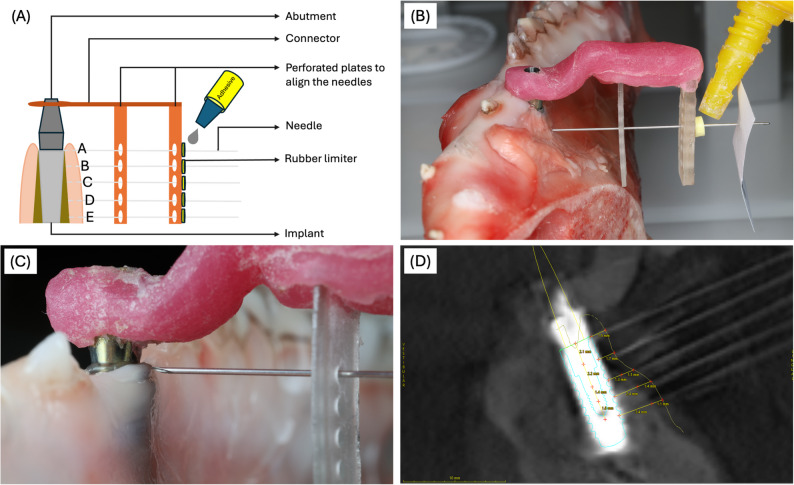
Mucosal thickness measurement using transgingival probing and cone*-*beam computed tomography. A Measurement device for transgingival probing (TP) and cone‑beam computed tomography (CBCT) with reproducible horizontal and vertical alignment at standardized points (A–E). B Fixation of the rubber limiter. C Needle in contact with the mucosa at point A. D Superimposition of optical scan and CBCT with mucosal thickness measurements in coronal slices at points A–E
